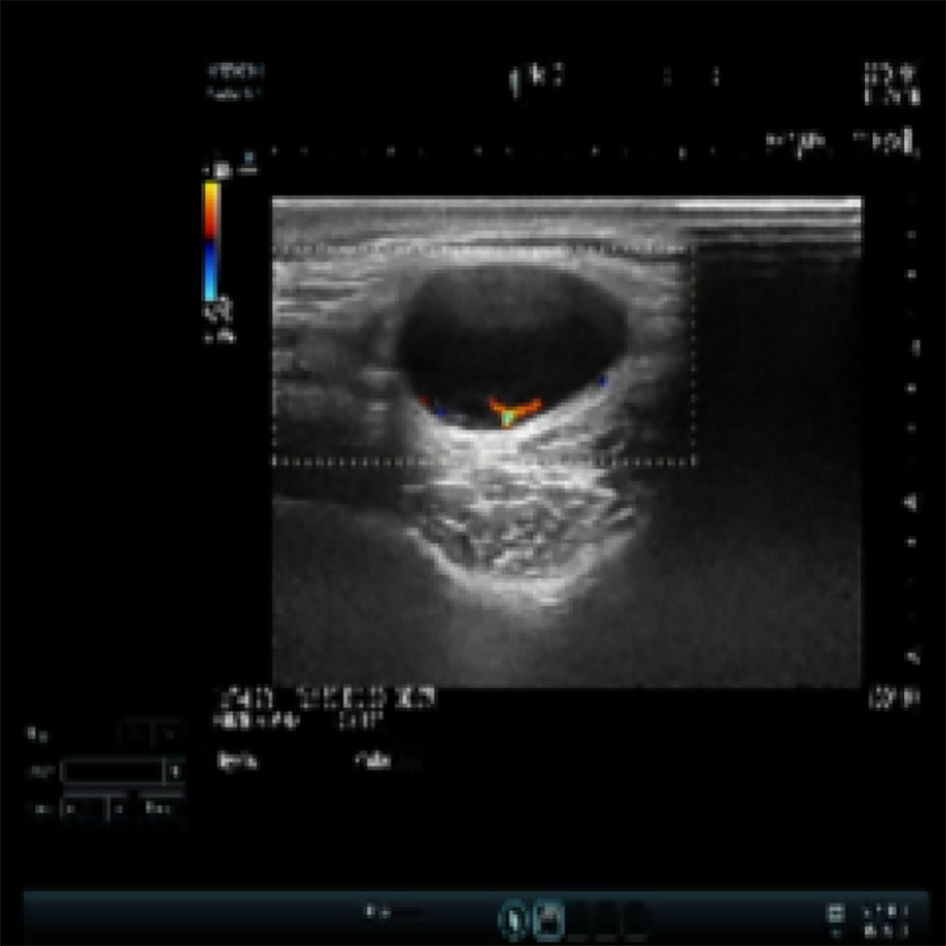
Body mass ultrasound of the patient.
